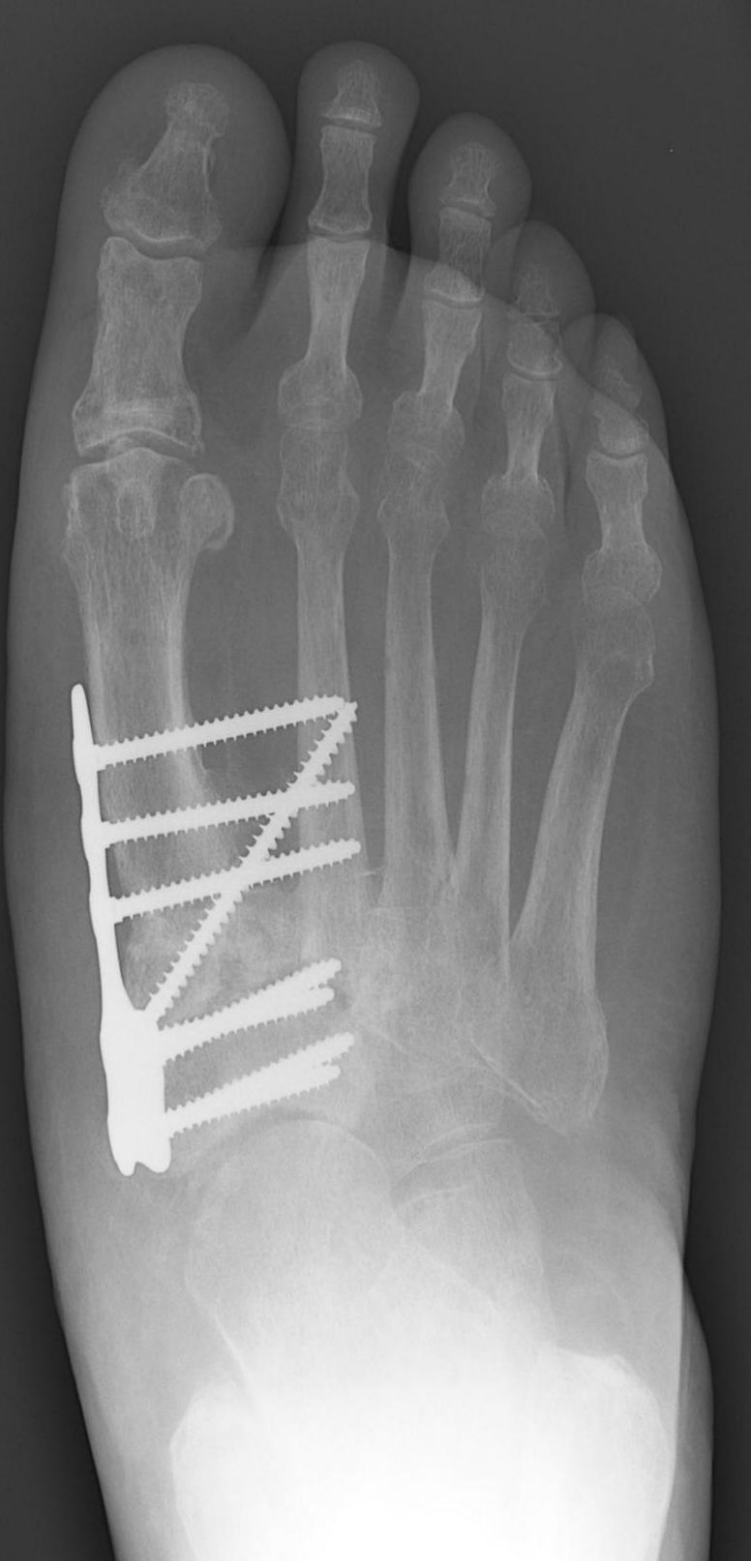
Final one year follow-up anteroposterior radiographic view showing anatomic alignment and consolidation across the arthrodesis sites.
